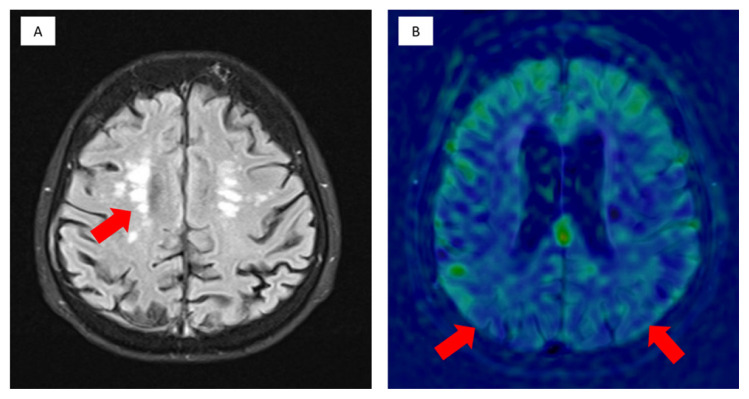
A 74-year-old lady with gradual memory impairment MRI (A) axial Fluid-attenuated inversion recovery (FLAIR) shows multiple foci of deep white matter hyperintensities, and (B) fused ASL perfusion with FLAIR axial imaging reveals significantly reduced cerebral blood flow of the bilateral parietal
